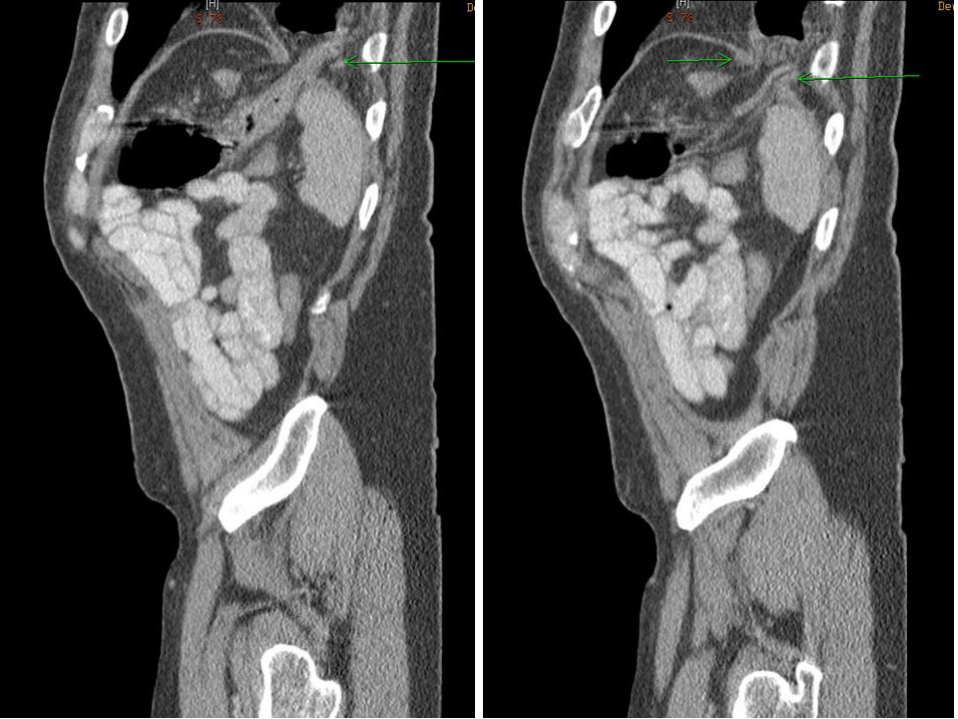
Saggittal CT showing disrupted left hemidiaphragm with herniation of bowel.
